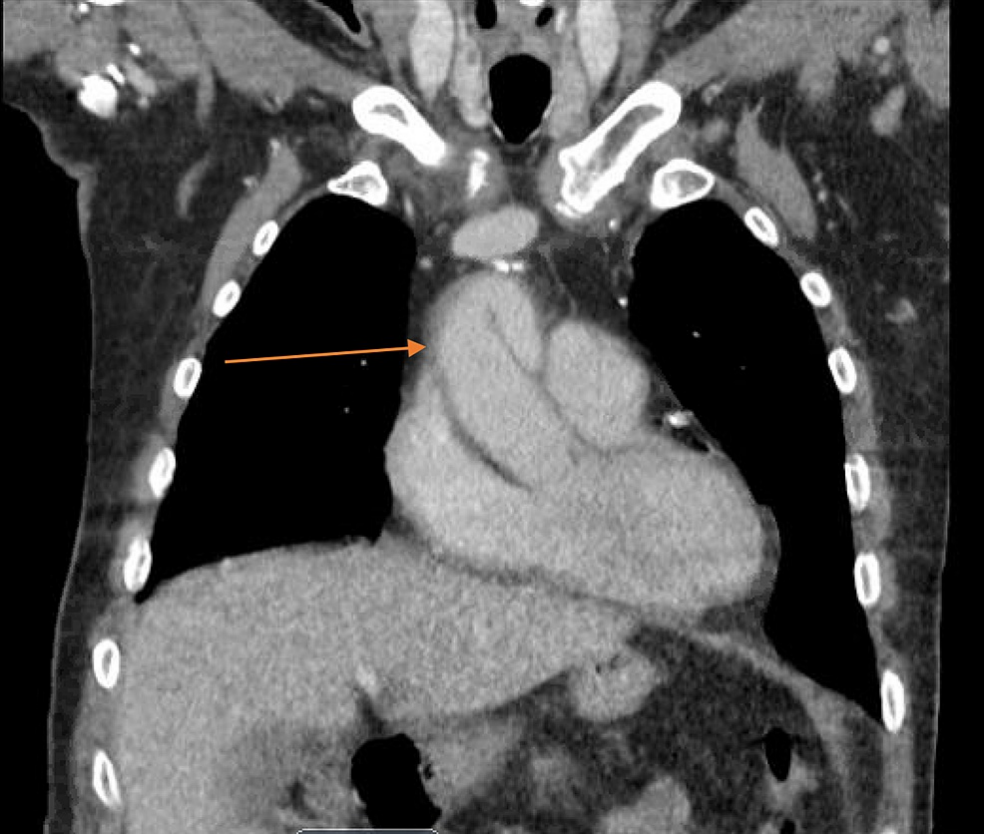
CT chest demonstrated a new 3.9 cm long segment of dissection of the ascending thoracic aorta, originating 3 cm distal to the aortic root. Arrow in figure pointing to dissection.
